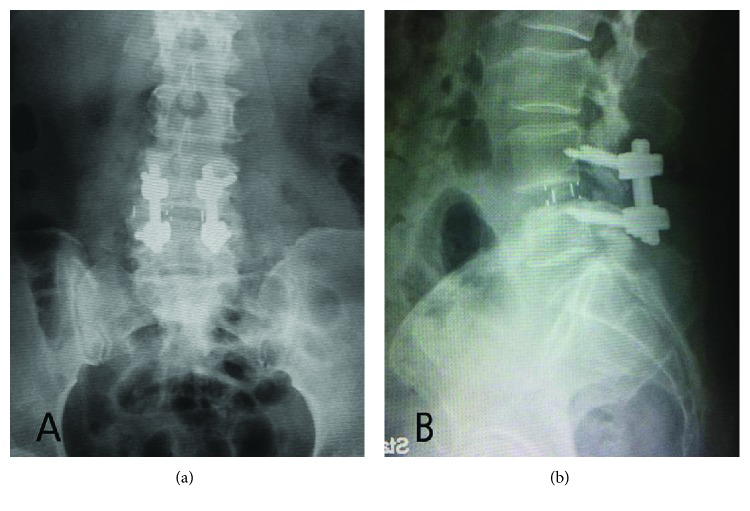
Postoperative plain films of lumbar spine AP and lateral (a and b) showed the MIS-OLIF PEEK cage was placed too deep over edge of right lateral of vertebral body.
